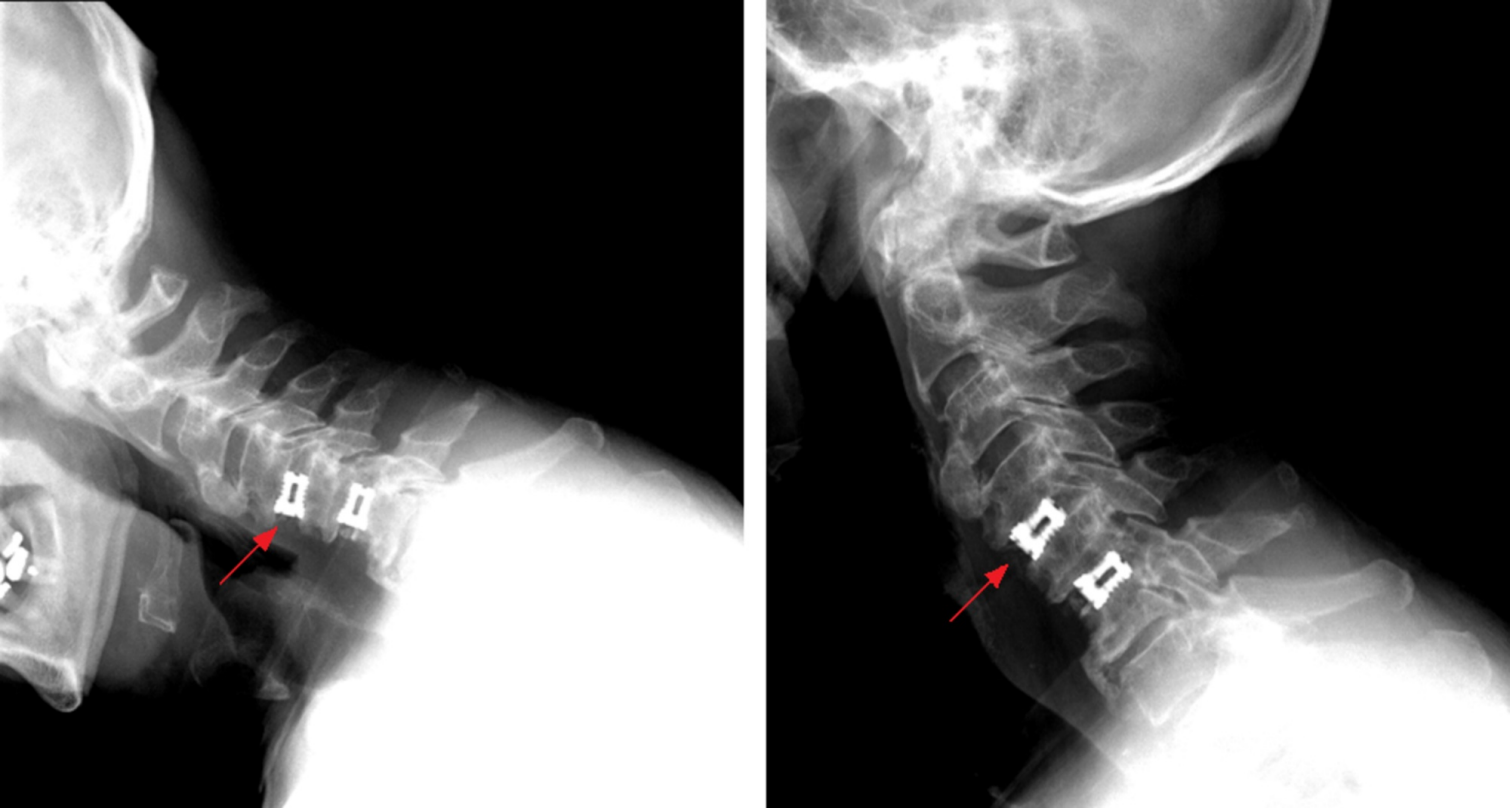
Dynamic lateral cervical X-ray imaging on six-week follow-up after VariLift-C® implantation. The wedge-shape of the implant (arrows) is identifiable and contributes to the correction of sagittal alignment. (Left) Flexion, (Right) Extension.
